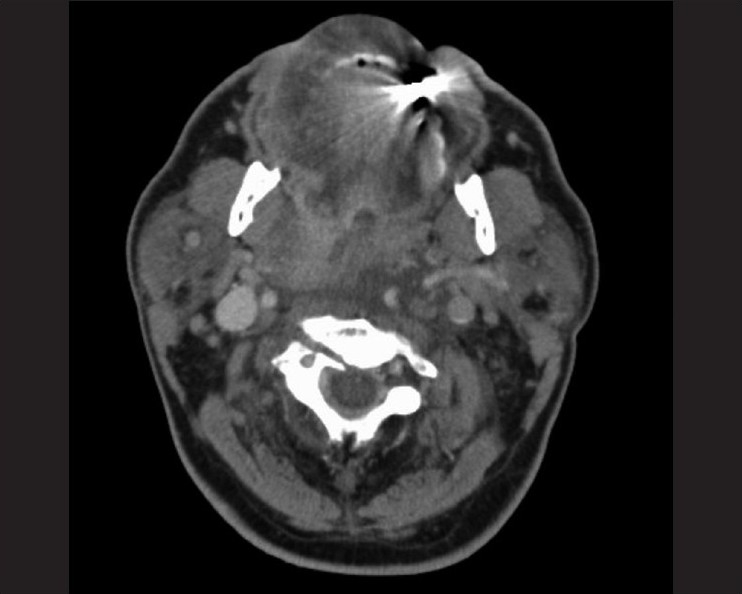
Transversal CT-scan of the C2-fracture
